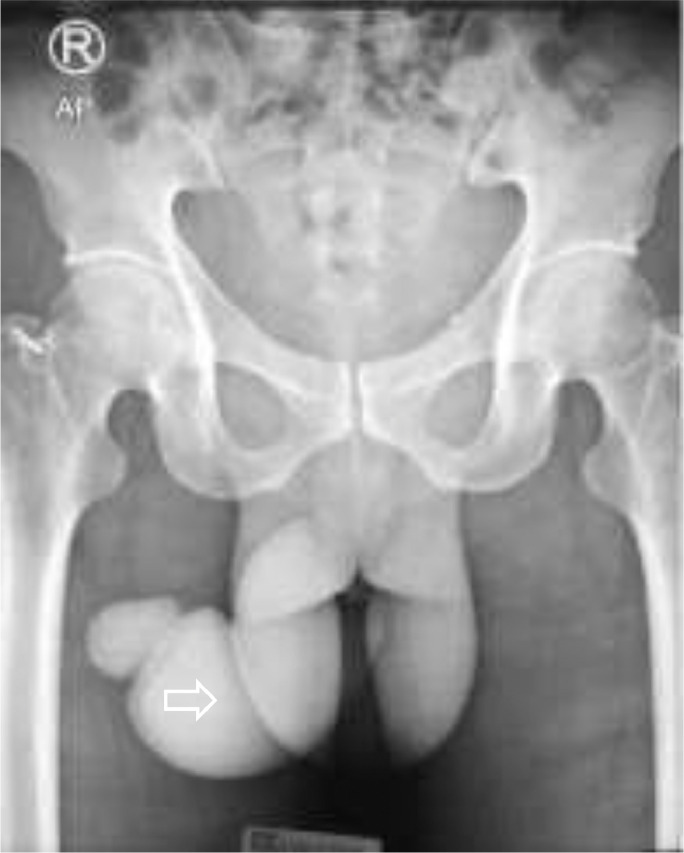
A plain abdominal radiograph showed soft tissue swelling in the penile region, with no diastasis pubic symphysis and opaque shadow in the urinary tract.
